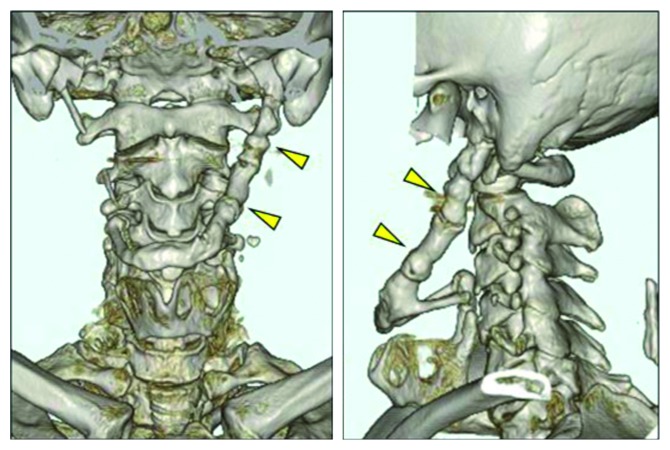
Three-dimensional reconstruction of a computed tomographic image taken preoperatively showing that the left styloid process was fused completely to the hyoid bone and had a total length of 70 mm (arrowheads). It was approximately 10 mm thick along almost its entire length.
